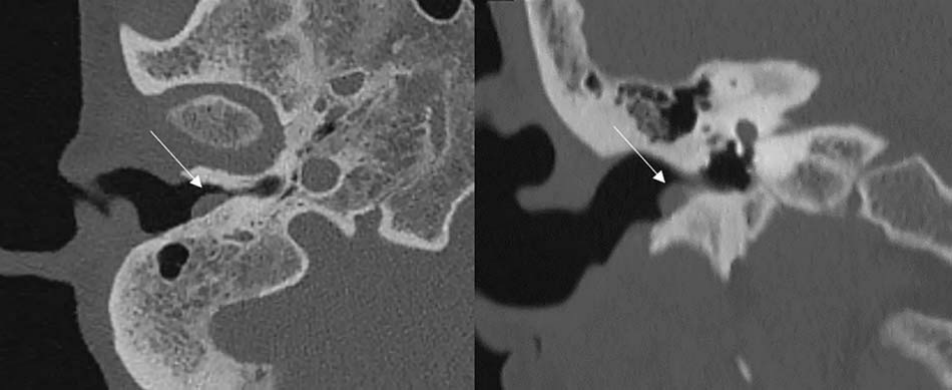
Axial and coronal CT scans showing a soft tissue mass (marked by the arrows) in the EAC without involvement of the tympanic membrane.
